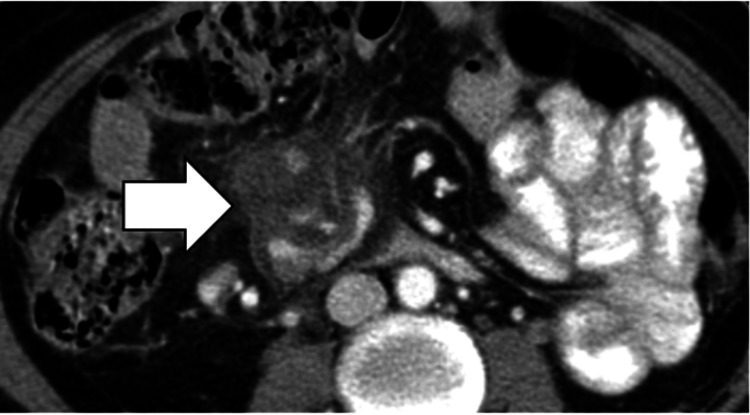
Lymphoma. CT shows a soft tissue mass at the mesenteric root, secondary to Burkitt’s lymphoma (white arrow).
